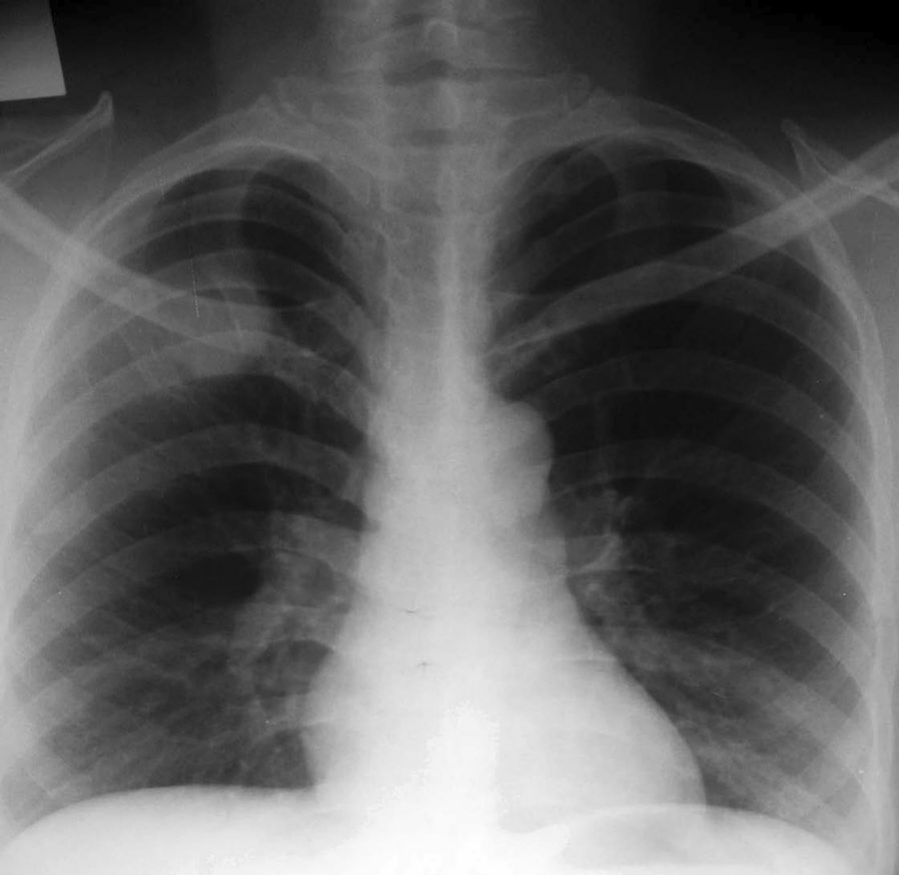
An antero posterior chest X-ray of the patient with a chest wall schwannoma
